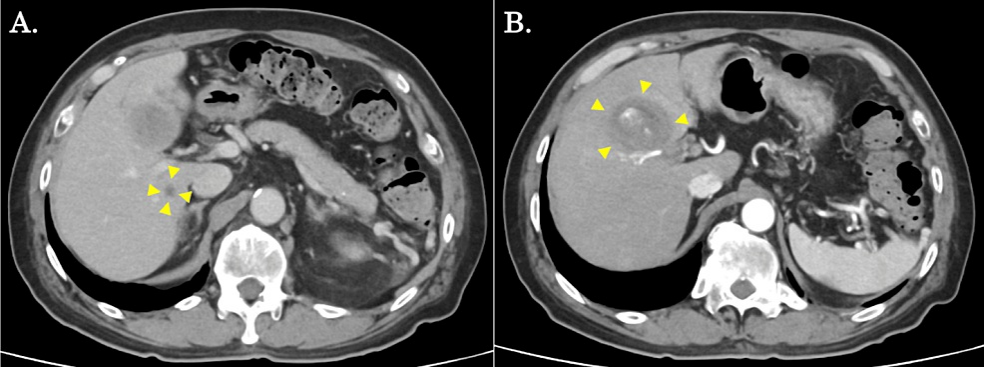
Contrast-enhanced CT findings after the biological therapy. (A) An axial contrast-enhanced CT scan revealed a reduction in the S7 tumor size of 9 mm (yellow arrows). (B) An axial contrast-enhanced CT scan revealed that the S4/5 tumor became slightly enlarged (47 mm in size) (yellow arrows).
